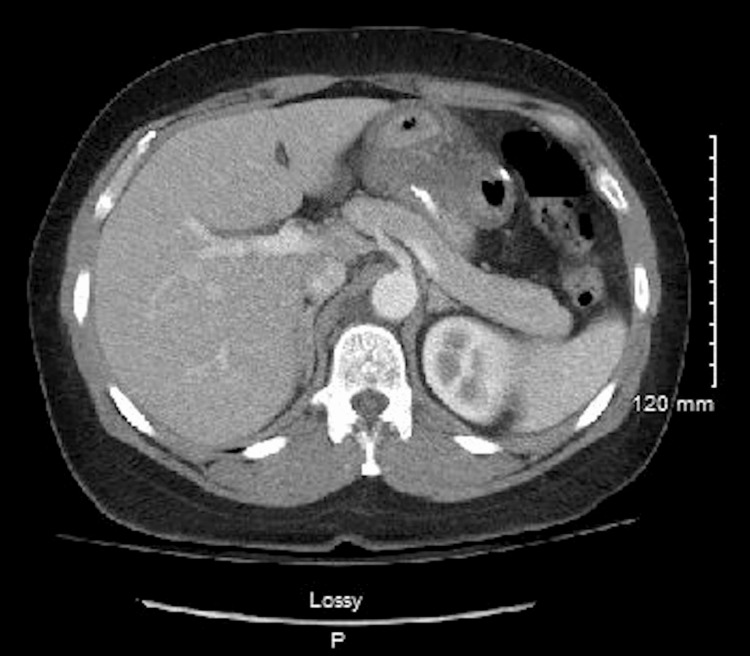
Axial contrast-enhanced CT image of the upper abdomen CT image demonstrates the Roux-en-Y anastomosis region, with circumferential wall thickening and submucosal edema involving the distal stomach and proximal jejunal loops, producing a “halo” appearance.
